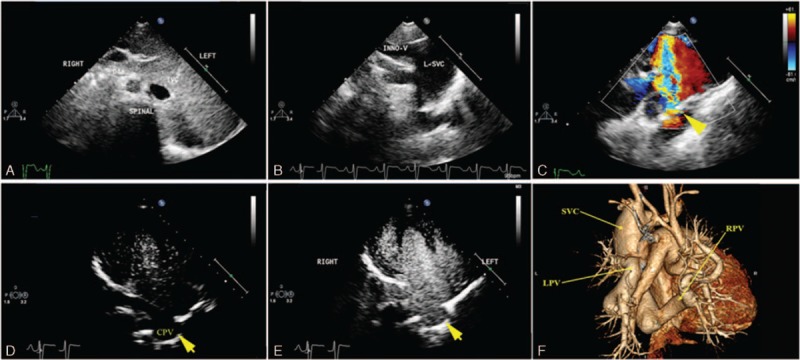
A 9-year-old girl with dextrocardia with anomalous pulmonary venous connections draining to aneurysmal left superior vena cava (LSVC) diagnosed by echocardiography, contrast echocardiography, and computerized tomography angiography (CTA). (A) The subcostal view shows the inferior vena cava located on the left side of the descending aorta. (B) The suprasternal view shows the LSVC dilated and connected to the innominate vein. (C) Using color flow Doppler, an abnormal accelerated flow spray into the LSVC was visualized. (D) Left-sided contrast shows no bubbles in the tubular structure (common pulmonary vein [CPV]) behind the atrium at first cardiac cycle. (E) After several cycles, bubbles appear in the CPV. (F) CTA shows the right atrium isomerism and left pulmonary vein confluence draining to the aneurysmal superior vena cava.
